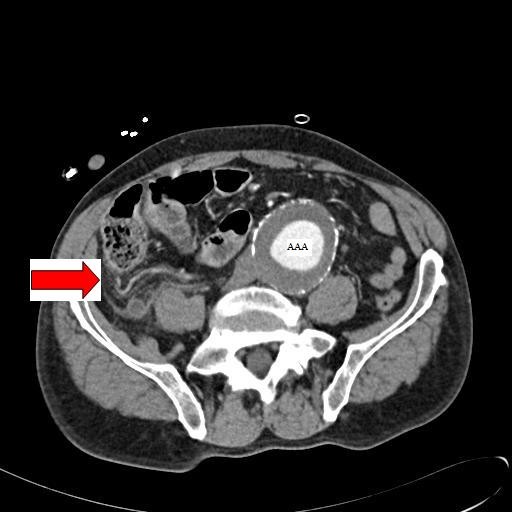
Abdominal CT image with contrast taken on the day of admission prior to the open Appendicectomy; showing an inflamed appendix (*marked by the red arrow*) and the AAA.
